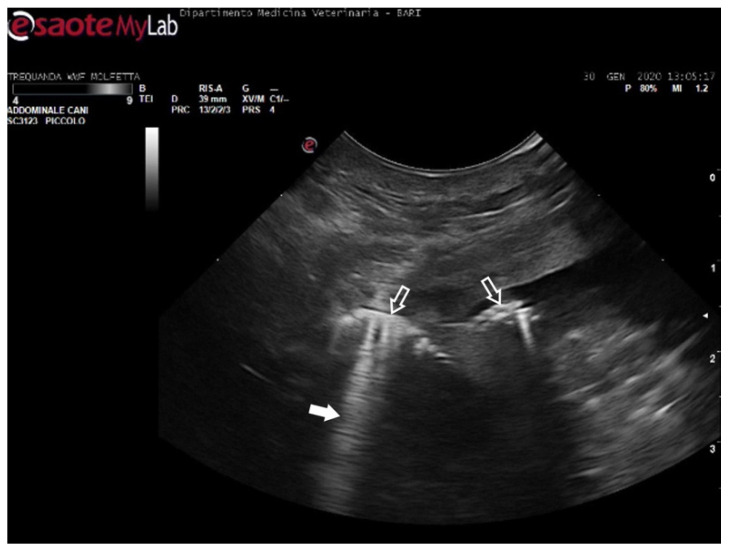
Ultrasound scan from the right prefemoral window revealing several clusters of large emboli attached to the hepatic vessels (empty white arrows). The artifacts generated by their presence (white arrow) make it challenging to visualize deeper structures.
